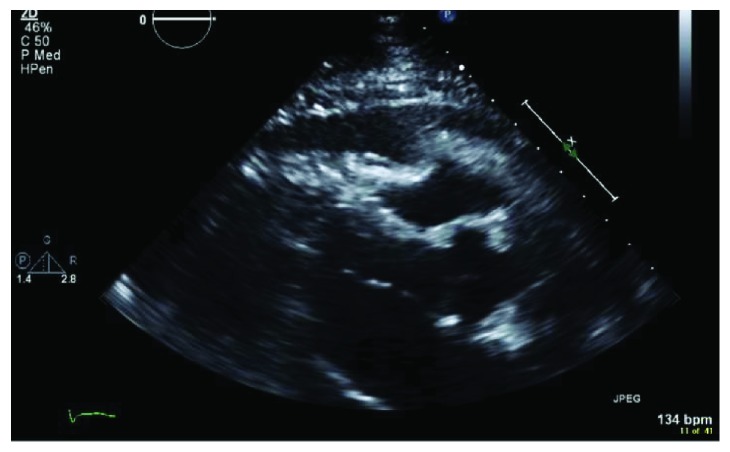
Transthoracic echocardiogram, parasternal long view, demonstrating large pericardial effusion and RV diastolic collapse.
